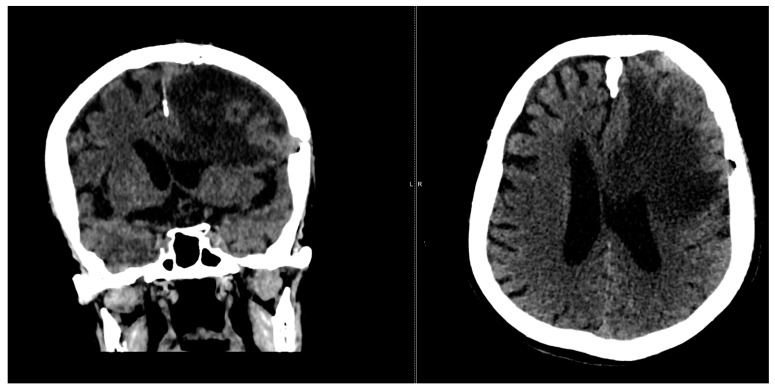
CT scan shows left paramedian frontal hypodensity, without contrast outlet.
